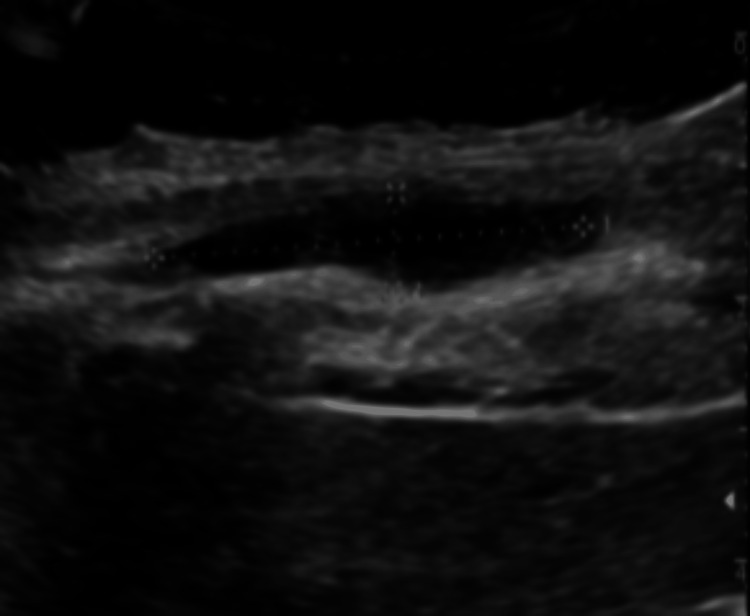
Decreasing chest wall lesion at one-month follow-up. Follow-up ultrasound of the right anterior chest wall at one month demonstrates a residual hypoechoic collection measuring approximately 3.3 × 0.7 × 0.7 cm (estimated volume ~1 mL). The collection is markedly reduced in size compared with the prior study.
